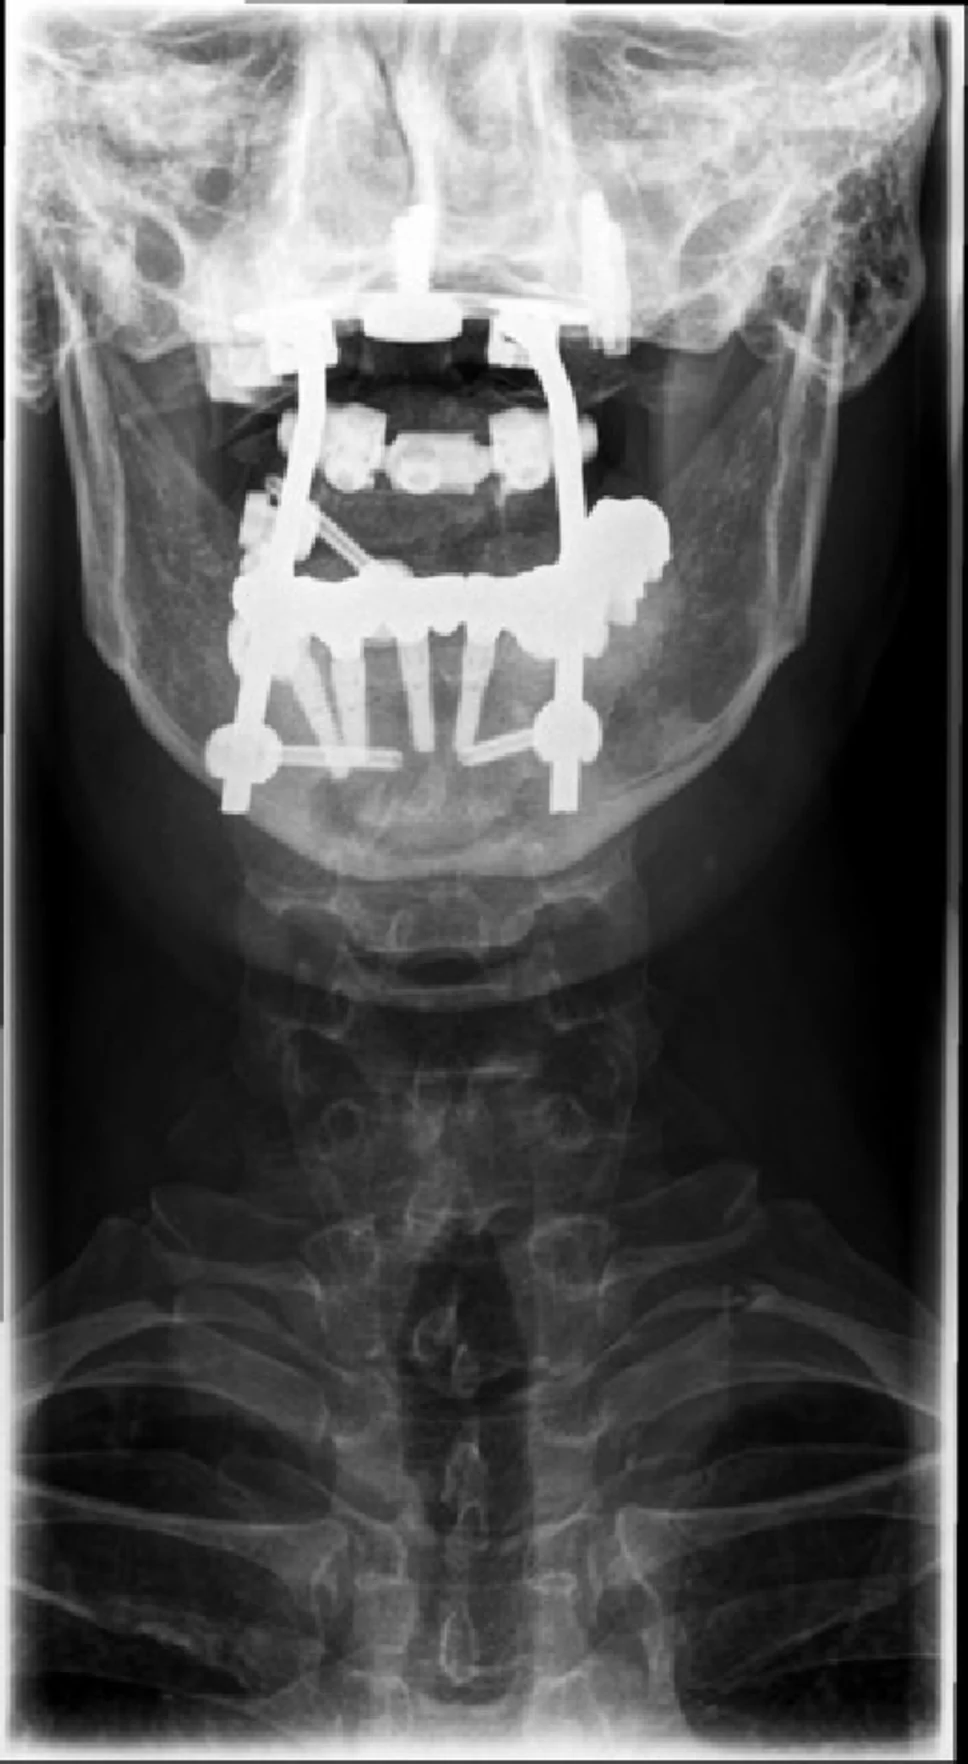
Anterior-Posterior X-ray of occipitocervical fusion (C0-C2-rt.-C3-C4) for C1 ring AO type B N0 fracture (= Gehweiler III), C2 AO type B N0 and Dens type III fracture (same patient as in figures 3 and 5–9. *C*,* cervical spine*
